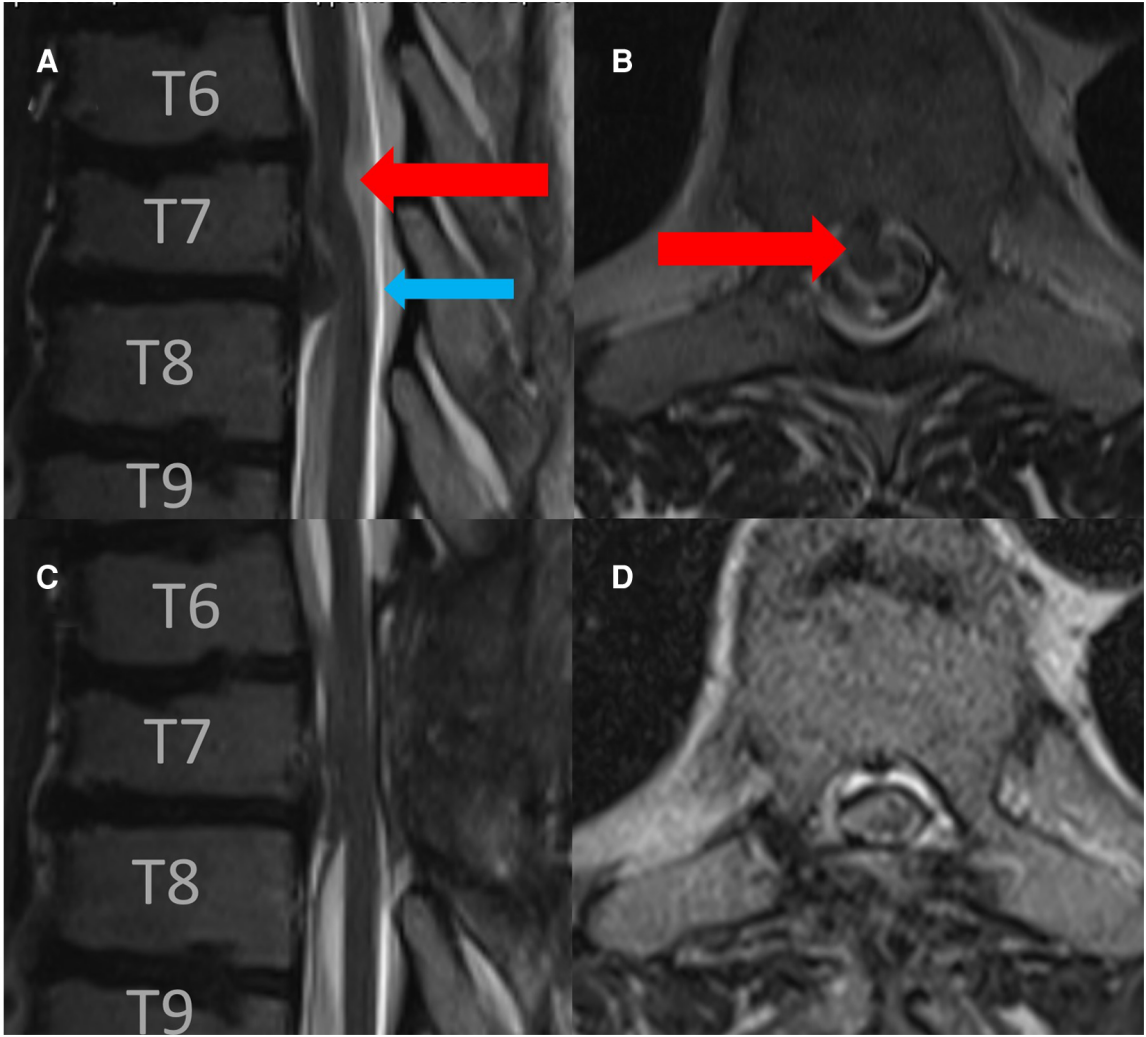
Preoperative sagittal (A) and axial (B) T2-weigthed MRI revealed a spinal cord herniation at the level of T 6/7 (read arrow) and disc herniation at the level of T 7/8 (blue arrow). Postoperative sagittal (C) and axial (D) follow-up T2-weigthed MRI after 3 months revealed the release of spinal cord herniation.
